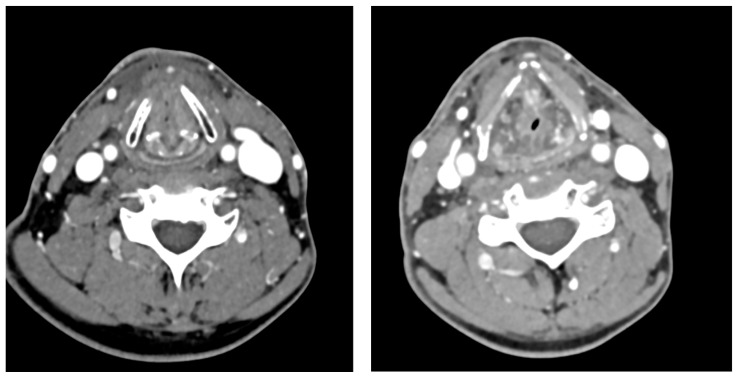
CT scan images after the treatment showing the tumor response.
